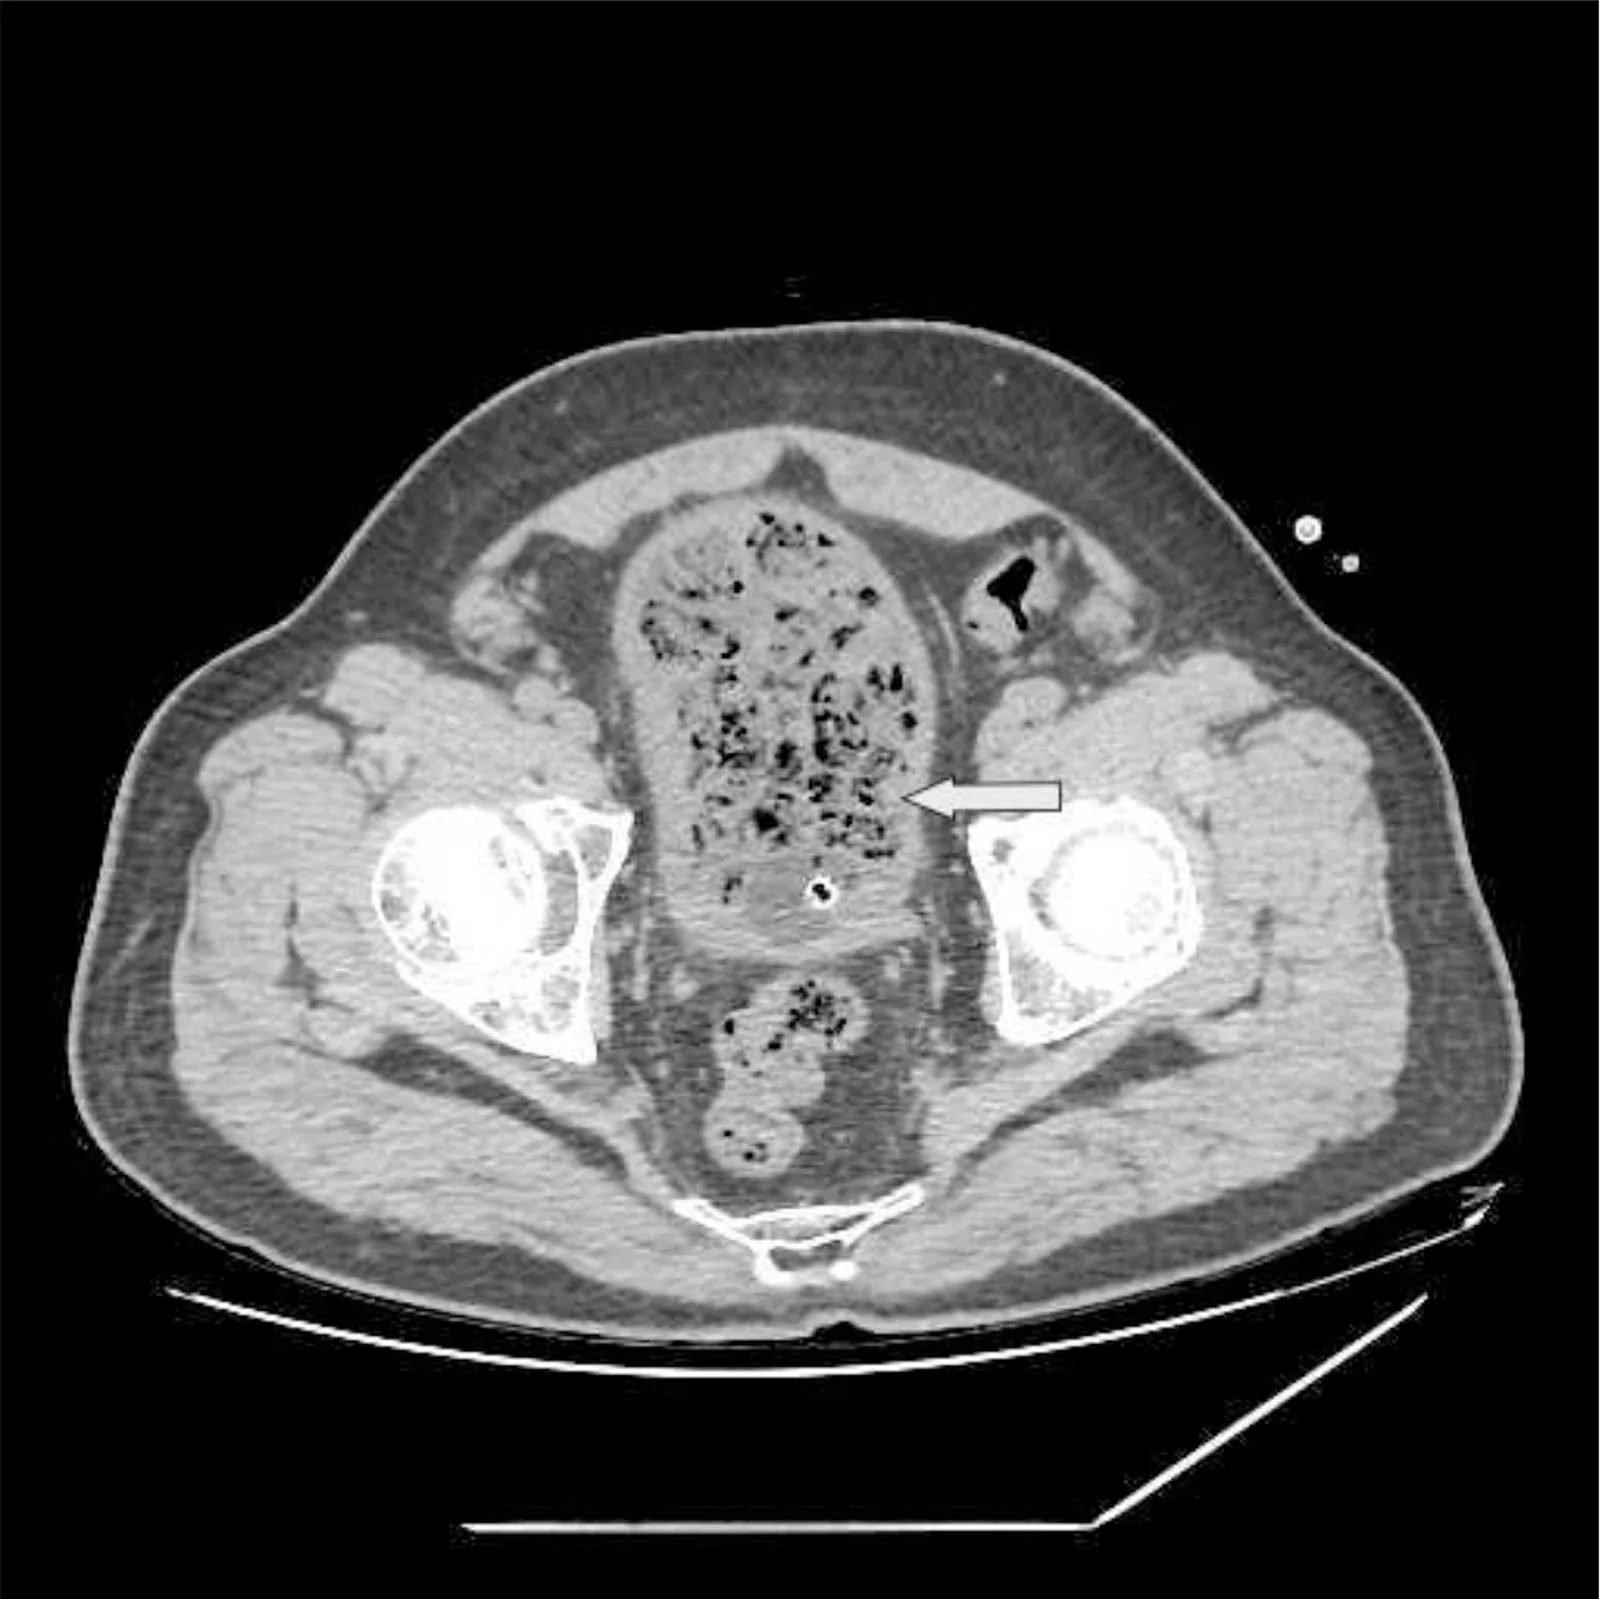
Follow-up axial noncontrast CT scan after 10 days of antifungal therapy demonstrates near-complete filling of the bladder lumen by enlarged, coalescing nodules (arrow), with resolution of the previously seen gas.
